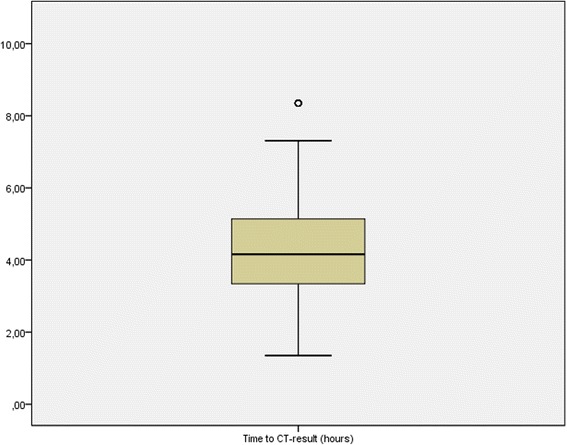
Time to CT-result (hours)
